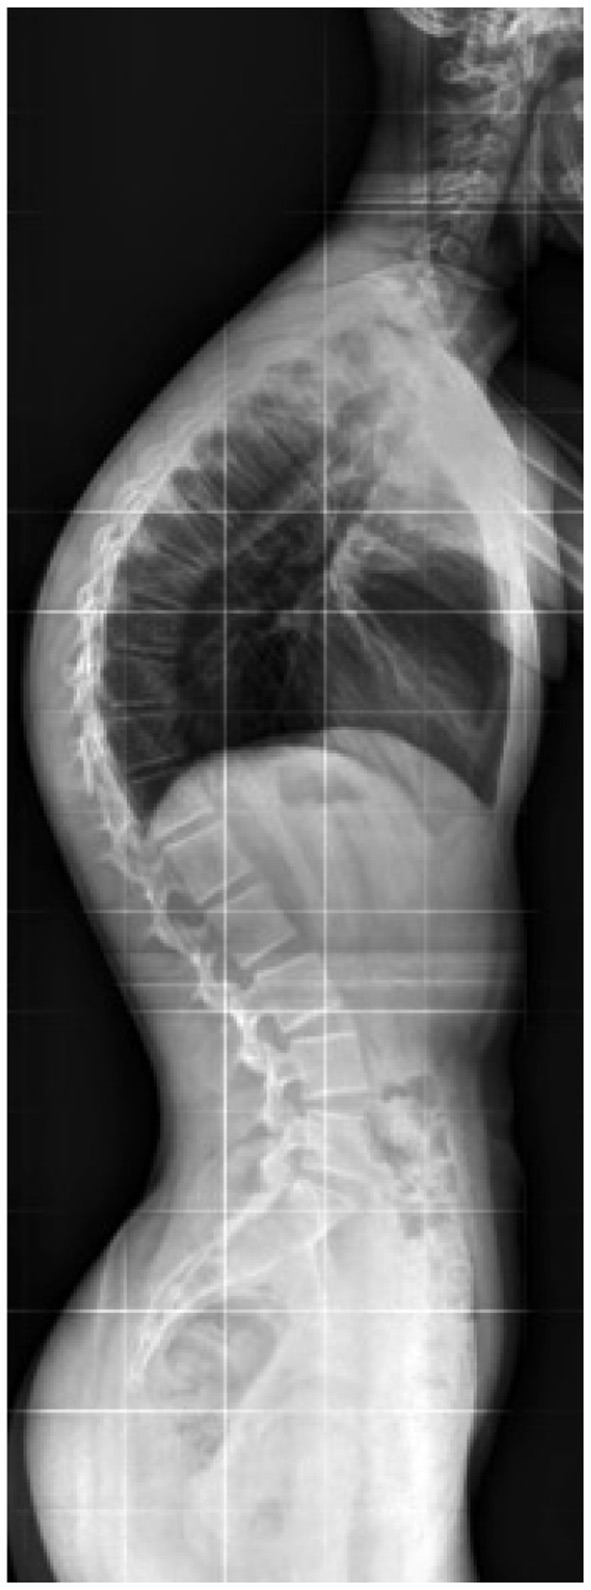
Profile x-ray of the spine.
